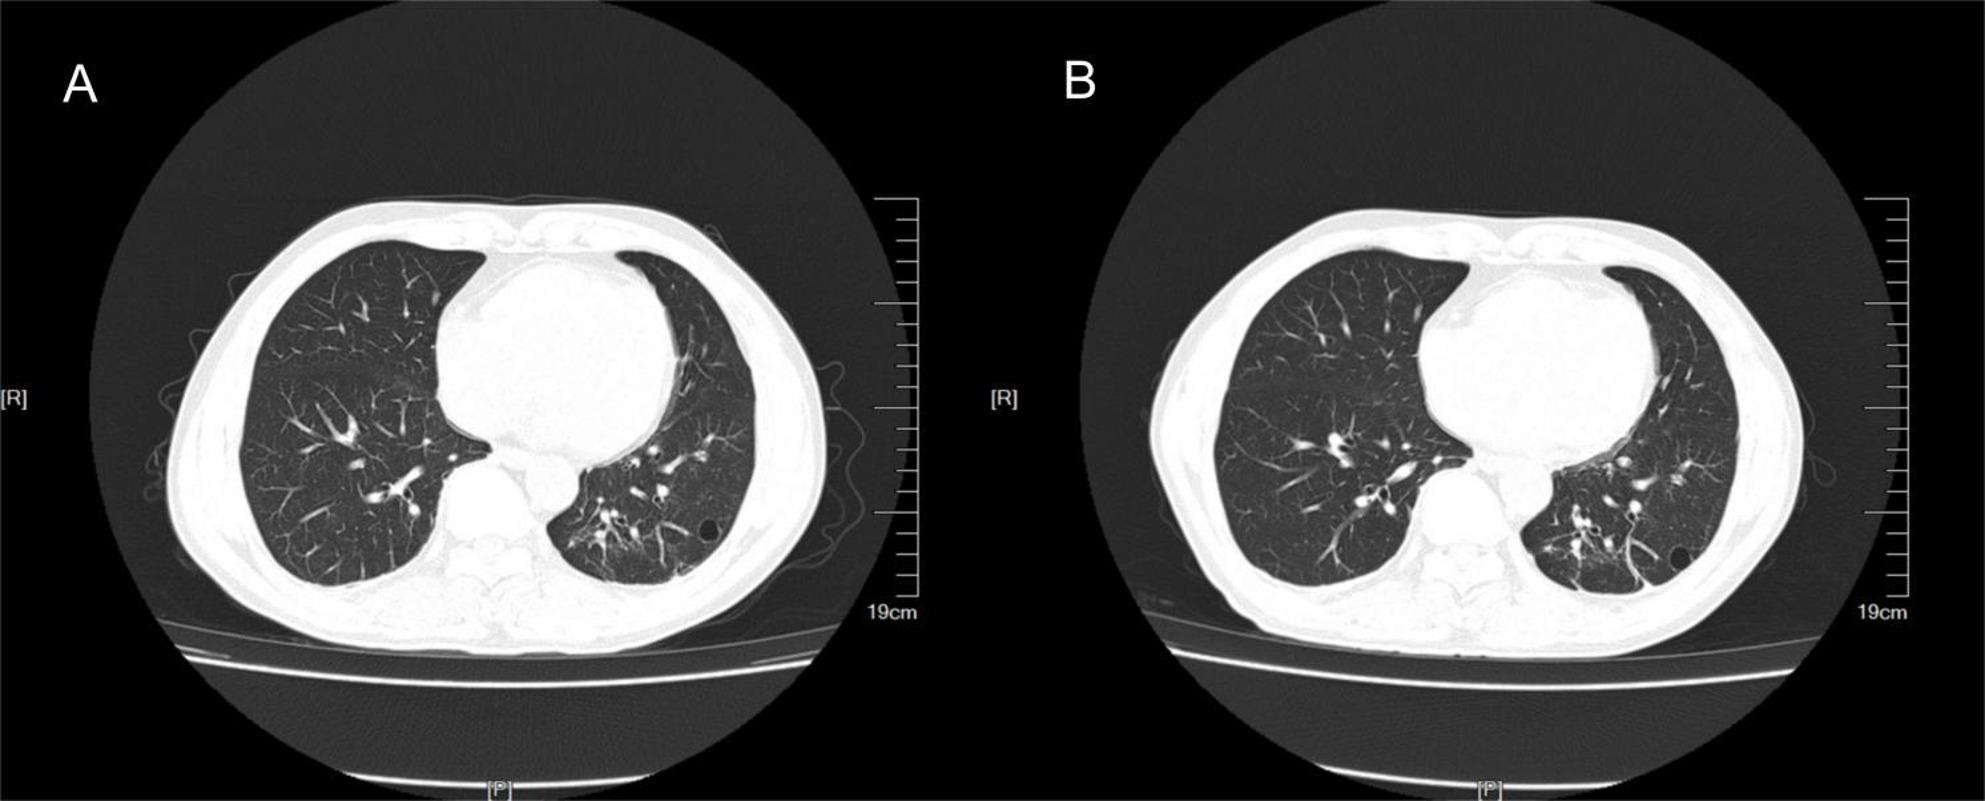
Lung CT revealed that the left inferior lobe of the lung presented a high-density shadow of a cable strip and a quasicircular transparent shadow. There was no obvious change before (A) or after (B) treatment
